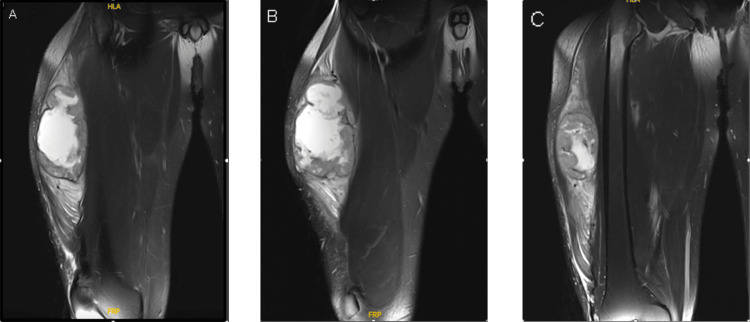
MRI T2 coronal cut (A) most anterior cut; (B) middle cut; (C) posterior cut This lesion measured about 8.7 x 8 x 12 cm
